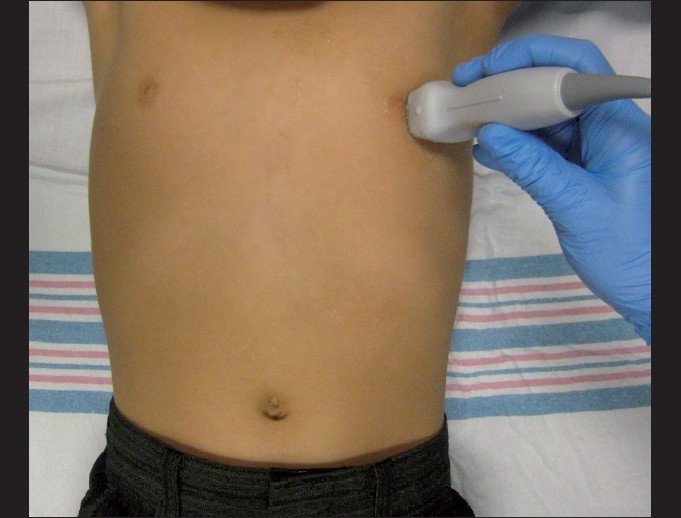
The apical 4-chamber view. The transducer is placed over the point of maximal impulse, oriented with the probe marker toward the patient’s right
